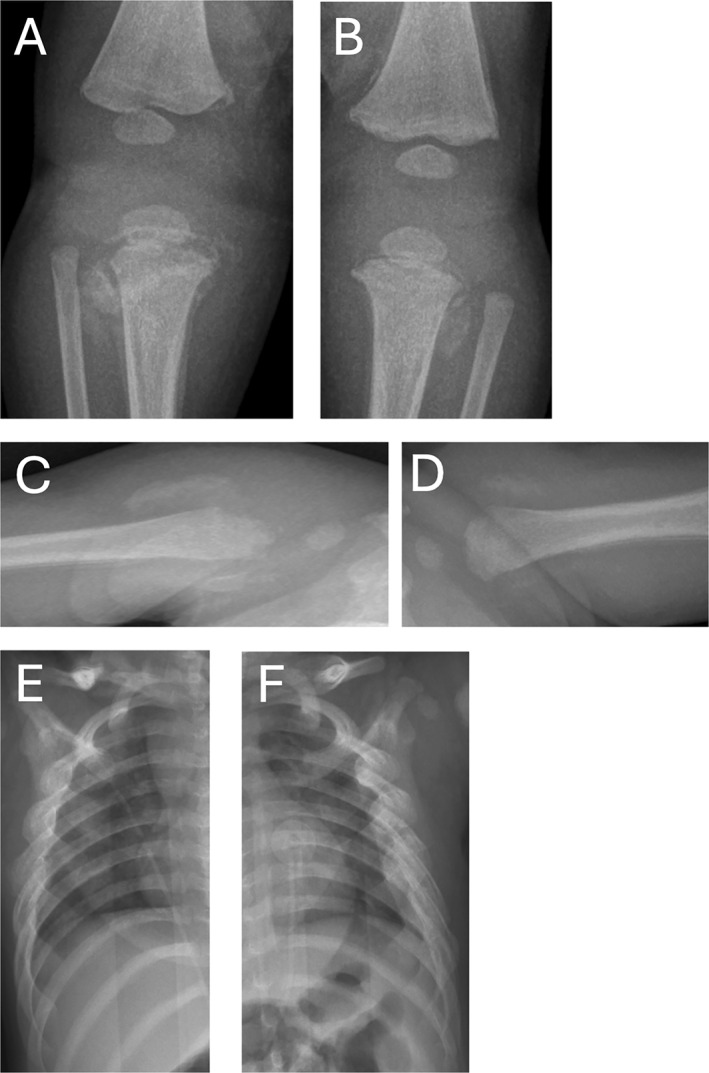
An 81‐day‐old male with witnessed reported physical abuse who presented before the pandemic. (A–D) Multiple fractures in varying stages of healing with radiographic features, such as corner and bucket handle metaphyseal fractures, and (E–F) bilateral clavicular fractures and multiple healing rib fractures.
